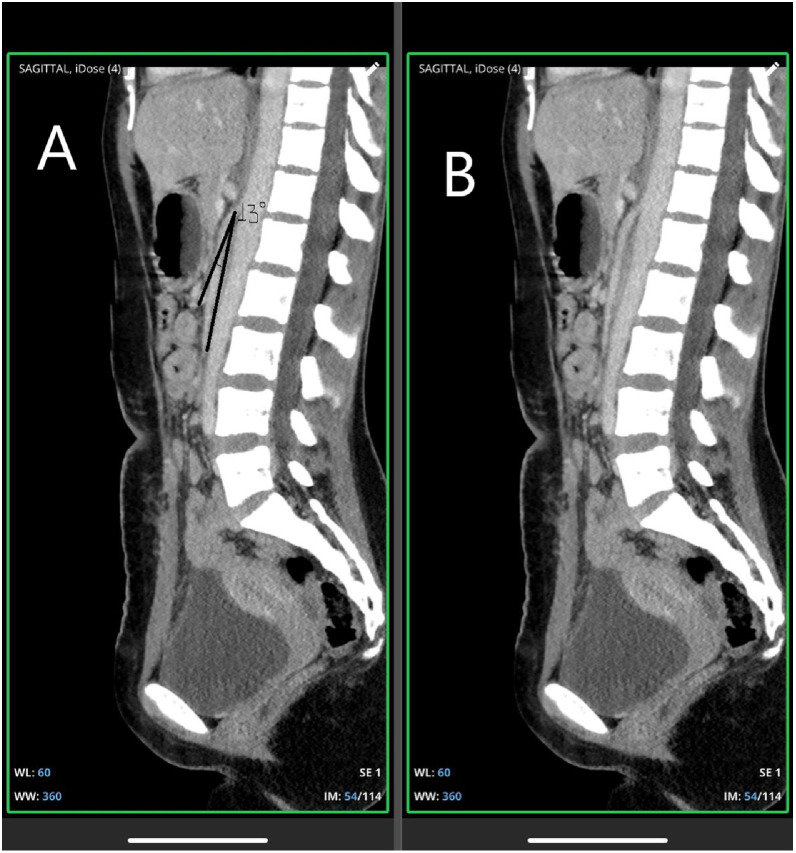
A-B: Saggital plane; CT scan of the abdomen with narrowed aorto-SMA angle (13^0^) (A) and clear version without annotation (B).
